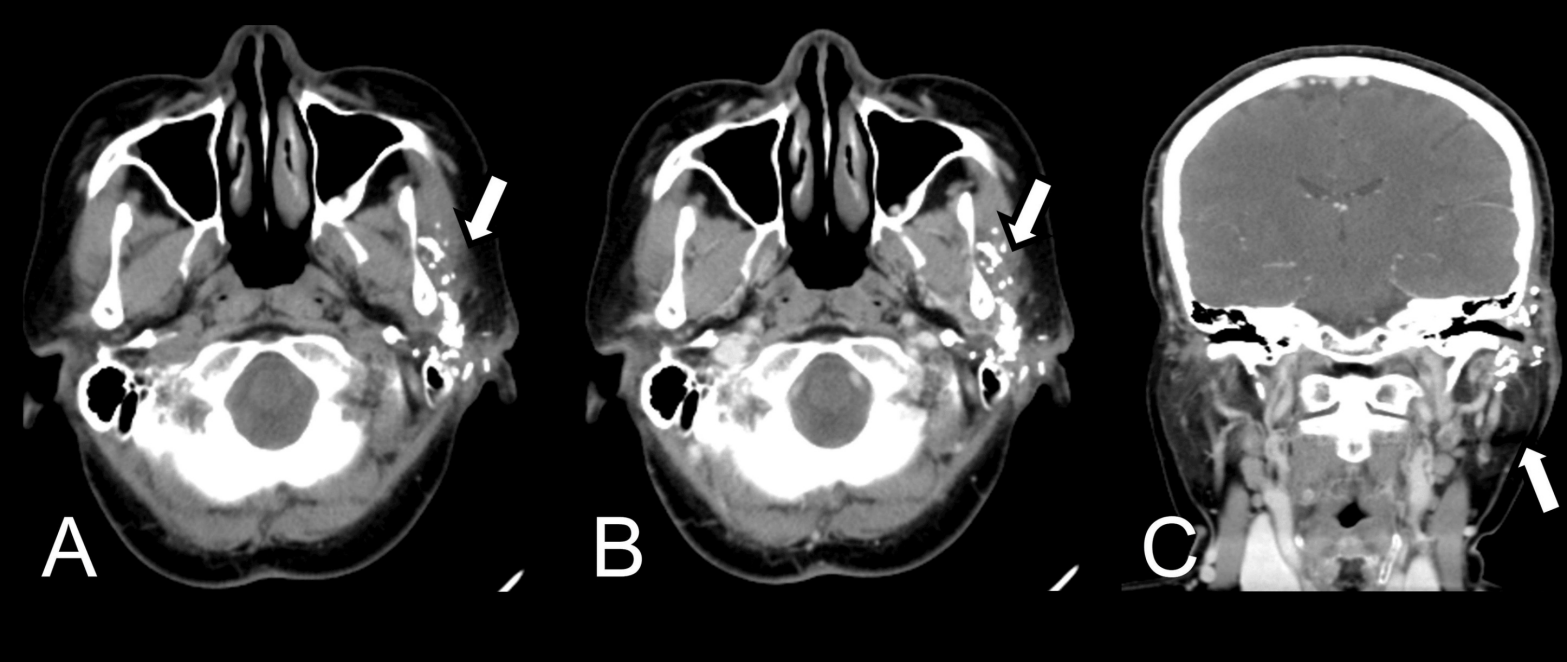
CT scan at the six-month postoperative follow-up. (A) Non-contrast and (B and C) post-contrast images show no obvious space-taking tumor identified. Focal skin thickening probably scar tissue and retained surgical materials. CT: computed tomography
